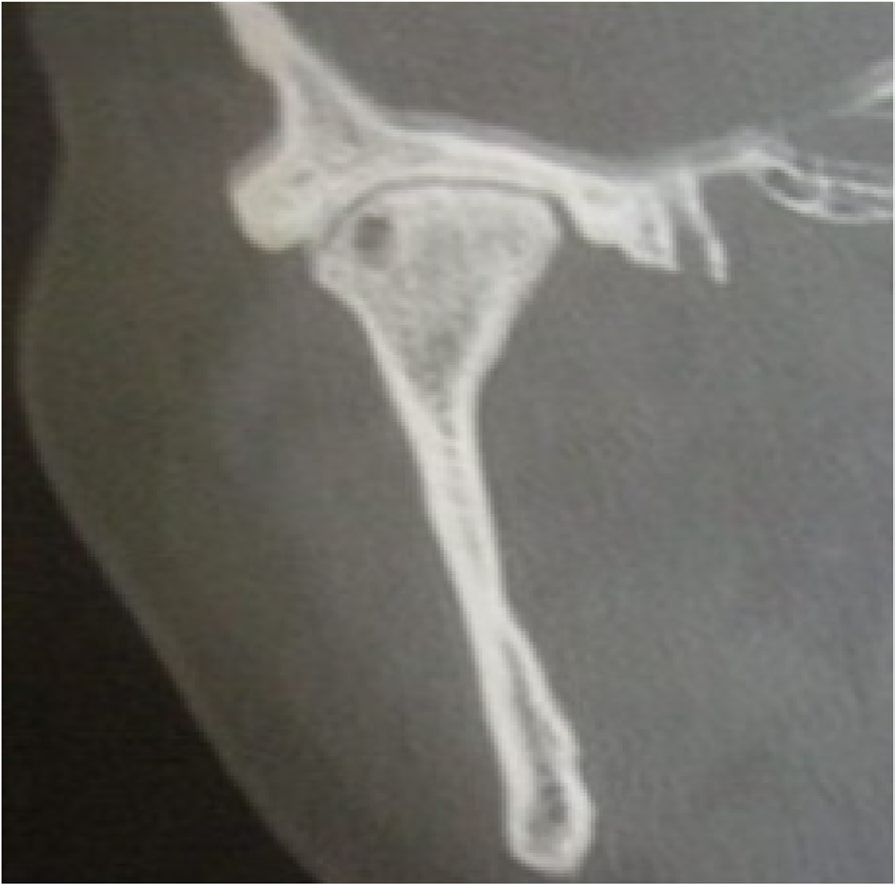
Coronal CBCT view showing Ely cyst in lateral pole of right condyle
